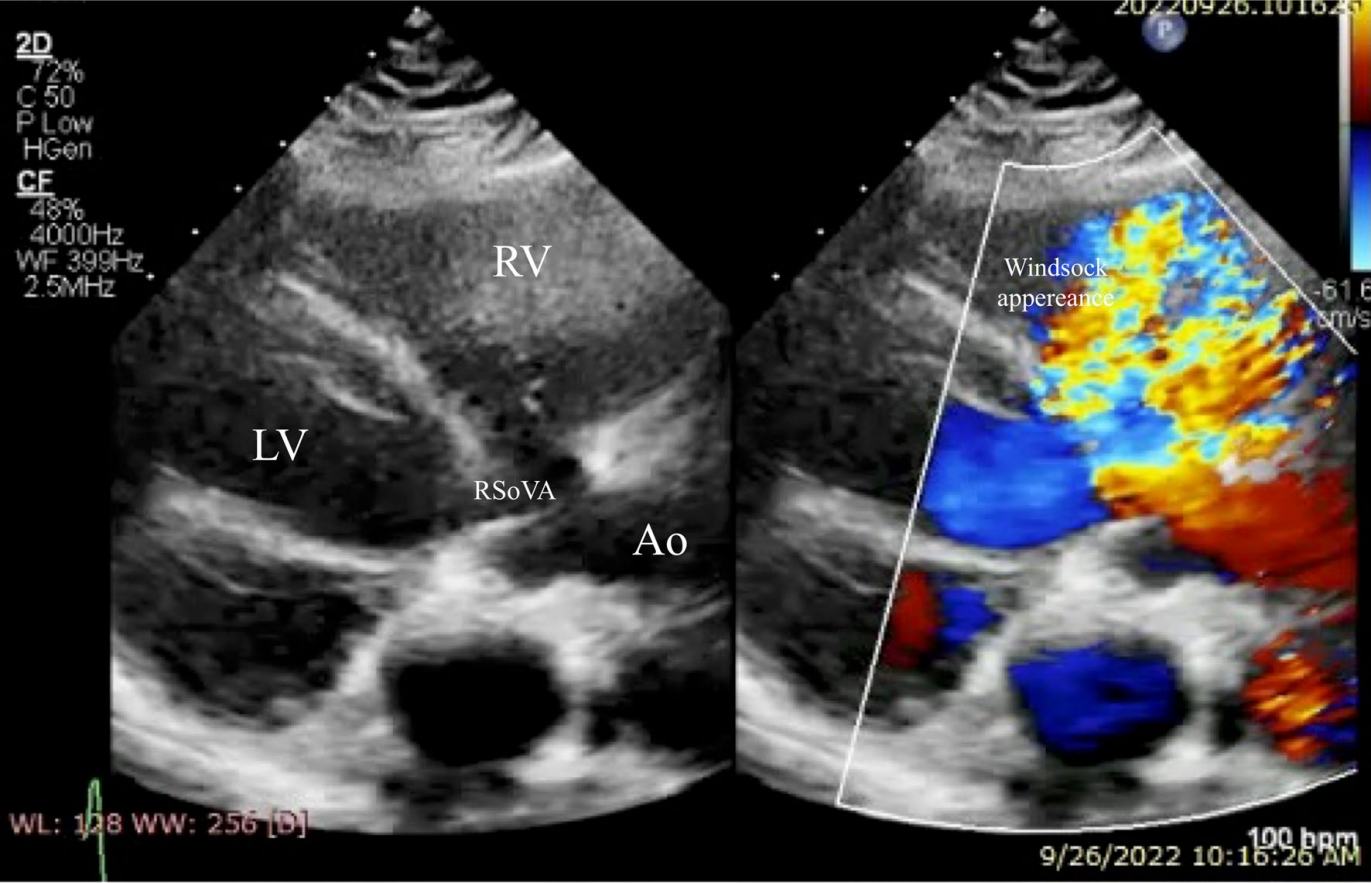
Transthoracic echocardiography identified a “windsock” appearance on parasternal long axis (PLAX) view. Ao = aorta; RV = right ventricle; LV = left ventricle; RSoVA: ruptured sinus of Valsalva aneurysm
